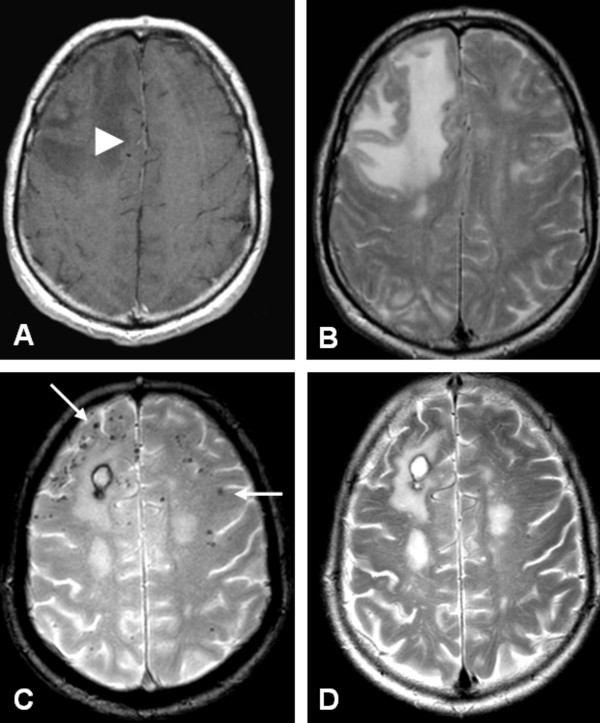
Axial MRI at presentation (A, B) and 3 months after treatment (C, D). A) Contrast enhanced, T1- weighted image shows low signal intensity of the right frontal lobe with minimal enhancement of the white matter (white arrowhead). B) T2-weighted image shows high signal intensity in the right frontal lobe. C) Gradient echo sequence shows subcortical 'black dots', consistent with microbleeds (white arrows), and a small postoperative hematoma after biopsy. D) T2-weighted image after treatment shows a decrease of high signal intensity in the right frontal lobe.
